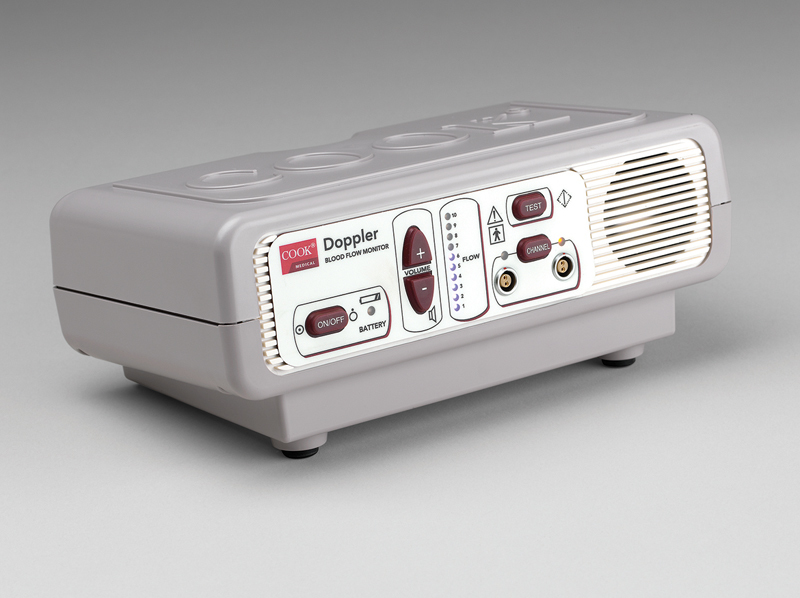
Doppler blood flow monitor.
